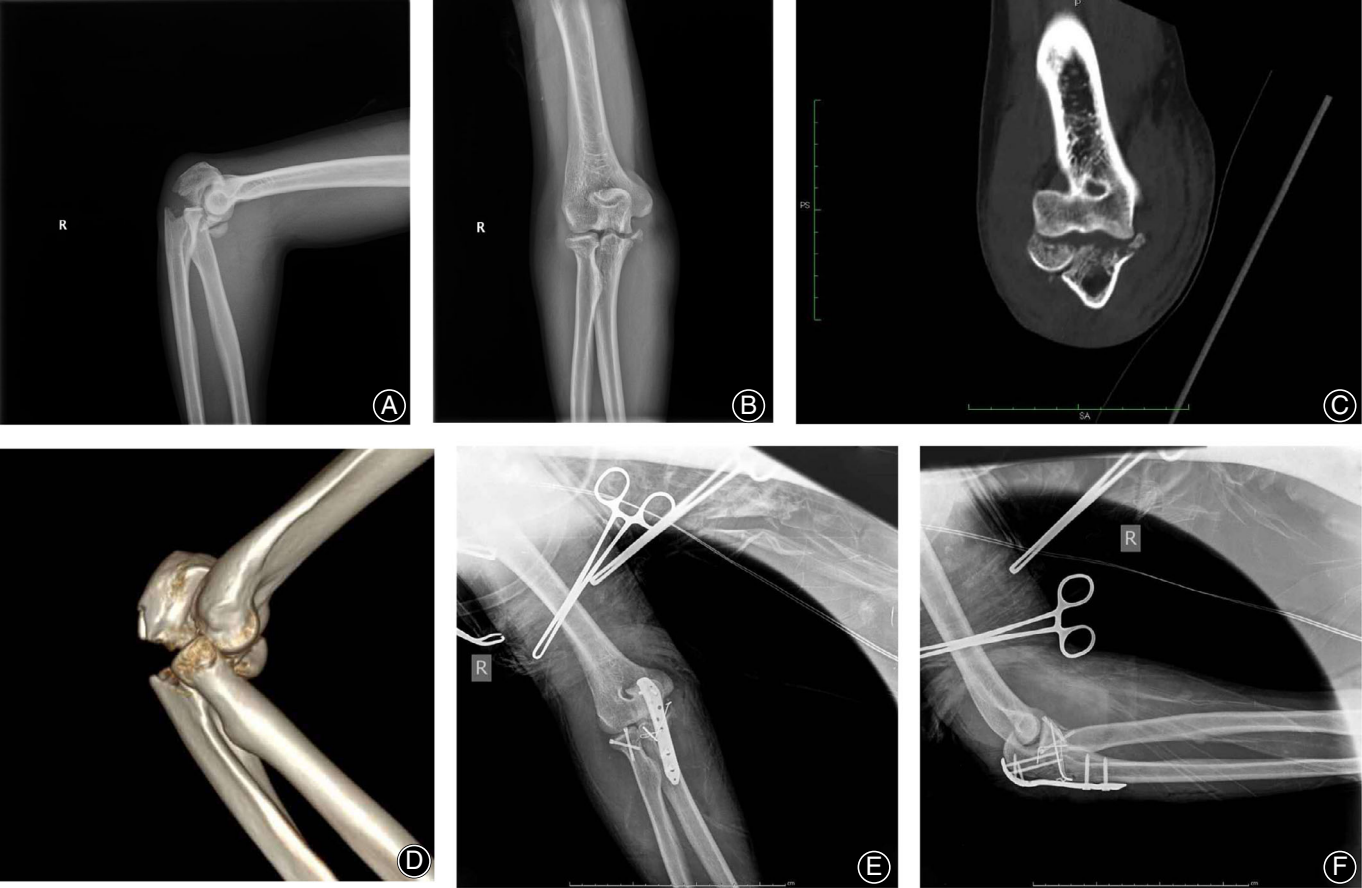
A 30‐year‐old man falling from 1 meter height and injured right dominant elbow. (A, B) Initial anteroposterior and lateral radiograph showed proximal ulna fracture and radial head fracture, with radiocapitellar dislocation. (C, D) A computed tomography image showed that the PRUJ saw no dislocation and no metaphyseal fracture was found. (E, F) The anteroposterior and lateral radiograph that were obtained following the operative treatment.
